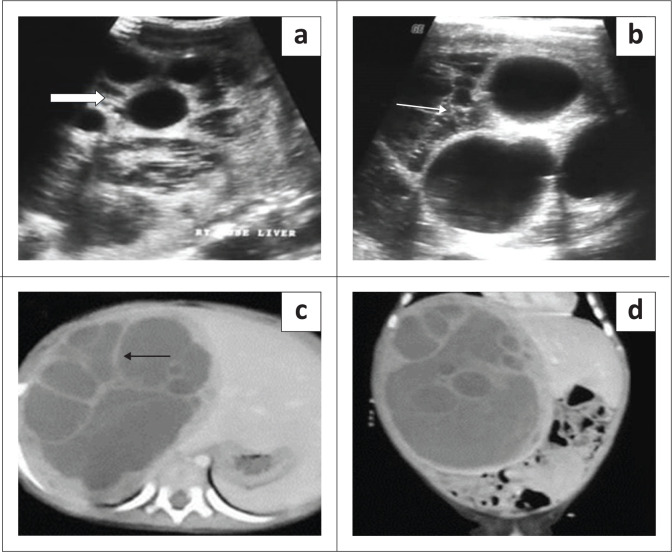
Mesenchymal hamartoma in a 9-month-old child. Transverse greyscale ultrasound (a) and (b) shows a multiseptated, cystic mass in the right lobe of liver (arrow in a) with no vascularity. Axial and coronal reformatted contrast-enhanced CT images of the upper abdomen in a different child show a large, peripherally enhancing, multiseptated, cystic lesion in the right lobe of liver with hepatomegaly. Enhancement of the septae (arrow in c) is also seen.
